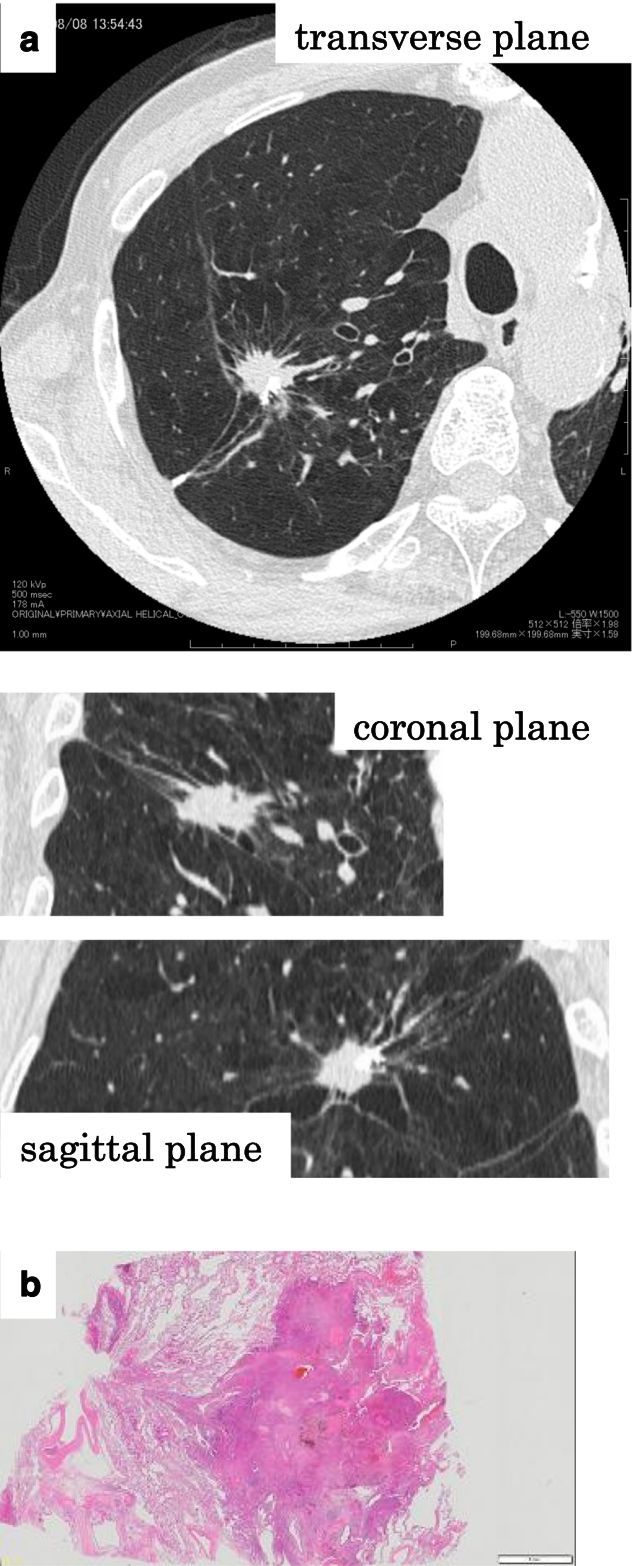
a Radiographic estimation by multi-planar reconstructions (transverse, coronal, and sagittal views) on thin-slice CT images (0.1-cm slice) before reoperation. b Pathologic features of the cut-end lesion of the residual right upper lobe, showing adenocarcinoma (macroscopic image)
